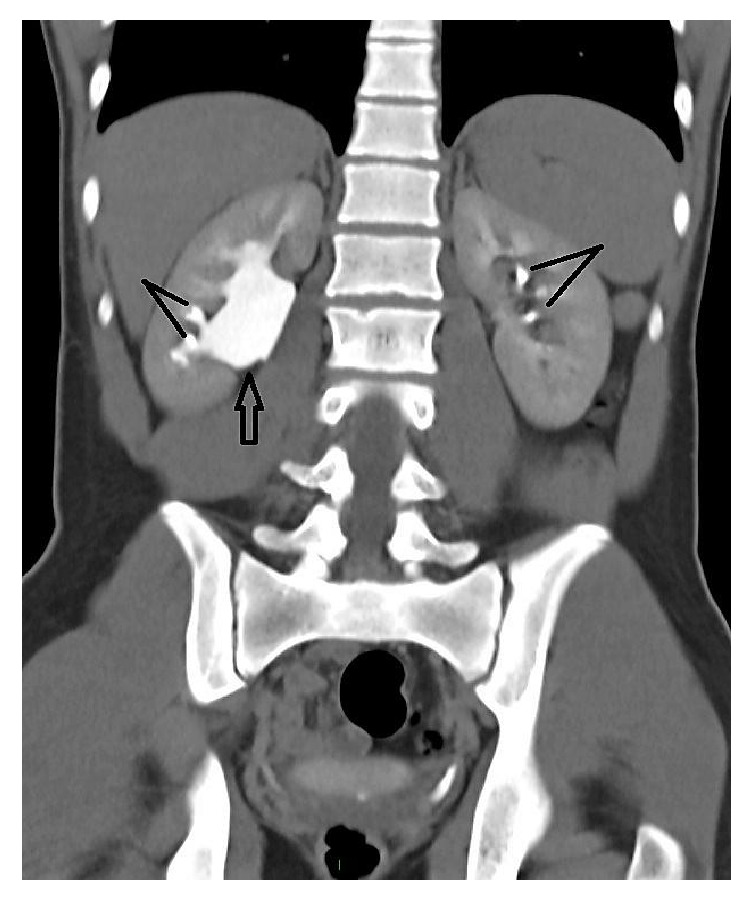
CT-scan of the abdomen and pelvis demonstrating moderately dilated right renal pelvis of 19 mm (headed arrow) and bilateral renal stones (dashes).
